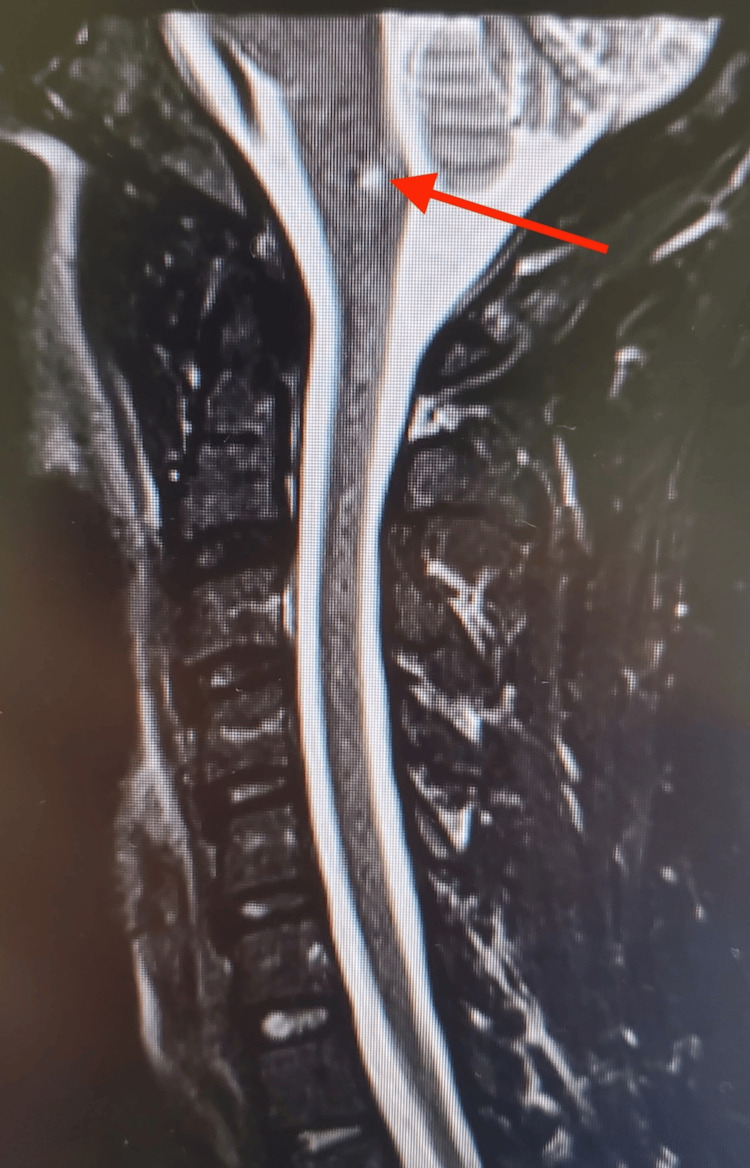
Sagittal MRI image of the brainstem demonstrating a hyperintense region at the site of infarction (red arrow).
